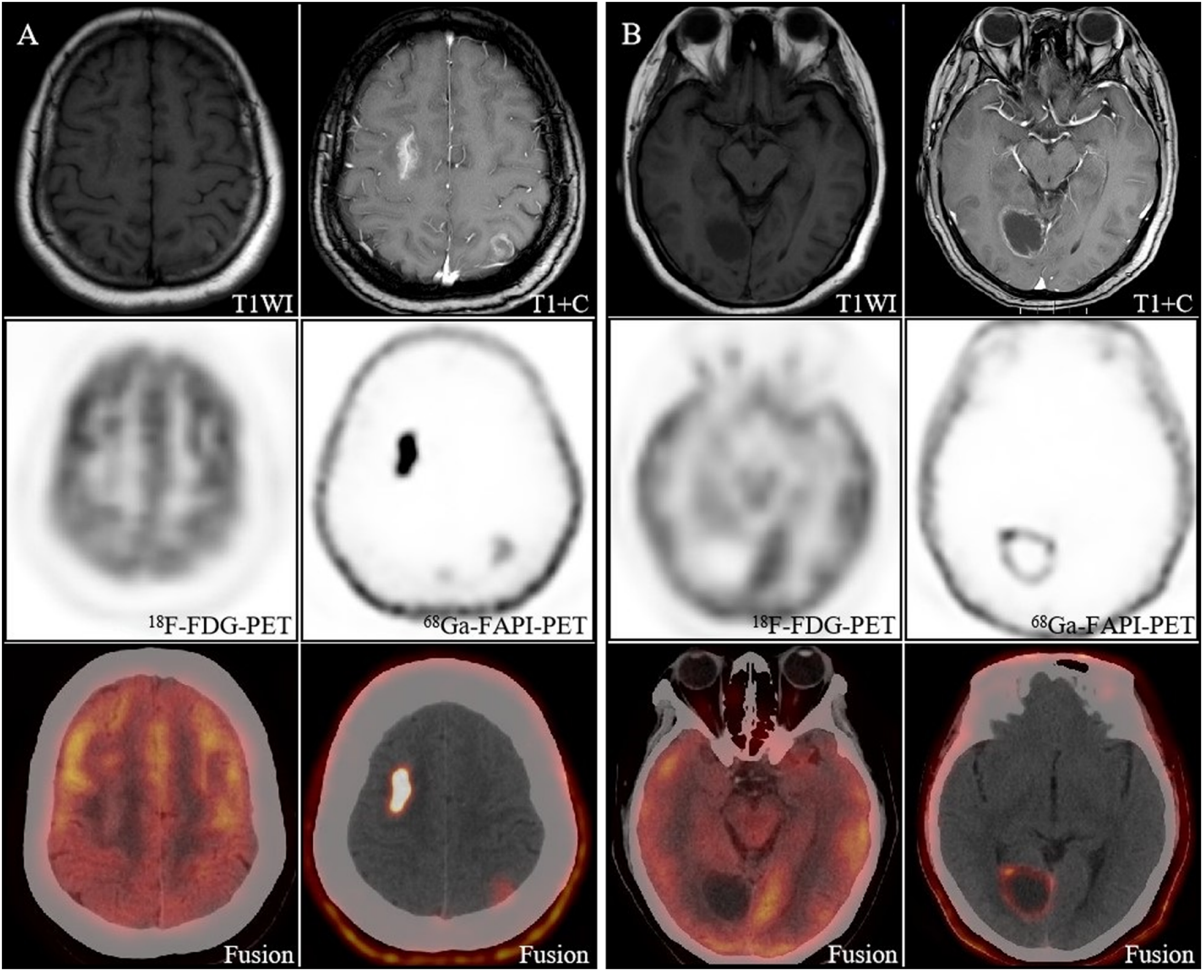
Image obtained in a 40-year-old woman with newly diagnosed lung cancer brain metastasis. (A) Brain metastasis in the right frontal lobe showed significant enhancement on MRI and showed intense [^68^Ga]FAPI activity (SUVmax 11.1). The lesion in the left parietal lobe showed ring-like mild enhancement on MRI and increased uptake on [^68^Ga]FAPI PET/CT (SUVmax 1.8). However, both lesions had no significant uptake on [^18^F]FDG PET/CT, and only the right frontal lobe lesion had morphological changes on CT. (B) The right occipital lobe lesion showed slight enhancement of the margins on MRI and increased uptake on [^68^Ga]FAPI PET/CT (SUVmax 4.8), which is still no uptake on [^18^F]FDG PET/CT.
